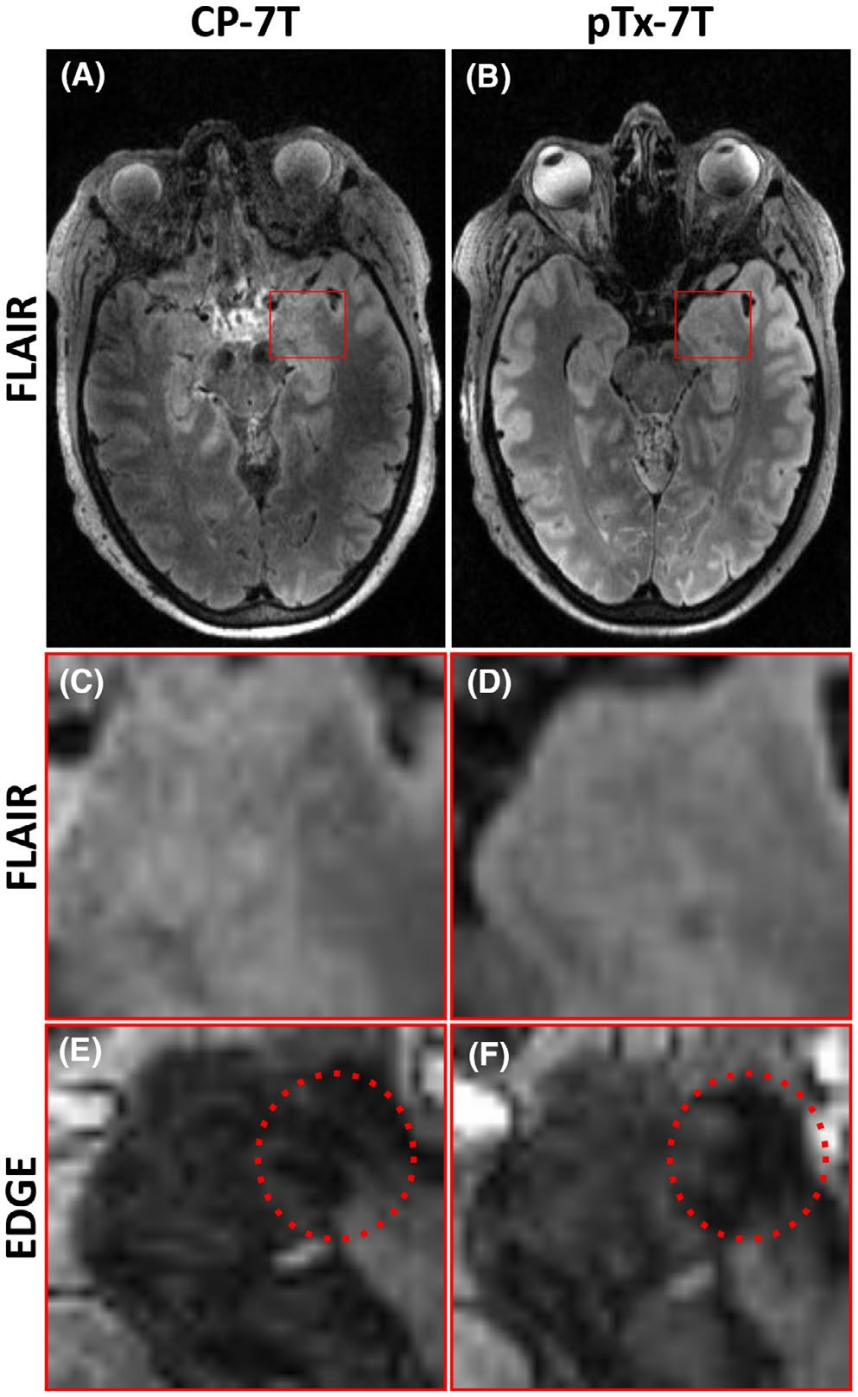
Patient 11 amygdala enlargement, visible on pTx but not CP images, due to improved uniformity of FLAIR sequence giving better definition of margins, and better clarity of EDGE contrast showing distension of lateral border.
